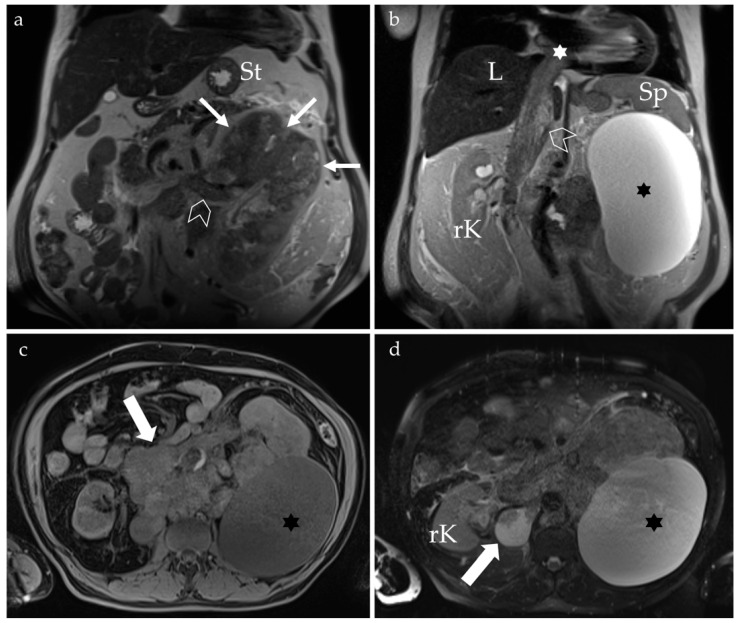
MRI examination of the abdomen, T2 weighted sequence in the coronal plane (a,b). A large left renal tumor (arrows), with tumor thrombus in the left renal vein (chevron, (a)) and inferior vena cava (chevron, (b)) extending into the right atrium (white star). A large left renal cyst is also noted (black star). T1 (c) and T2 (d) weighted image (WI) with fat saturation in the axial plane with arrows pointing to multiple retroperitoneal lymph node metastases; the left renal cyst is also depicted (black star). L = liver, Sp = spleen, St = stomach, rK = right kidney.
